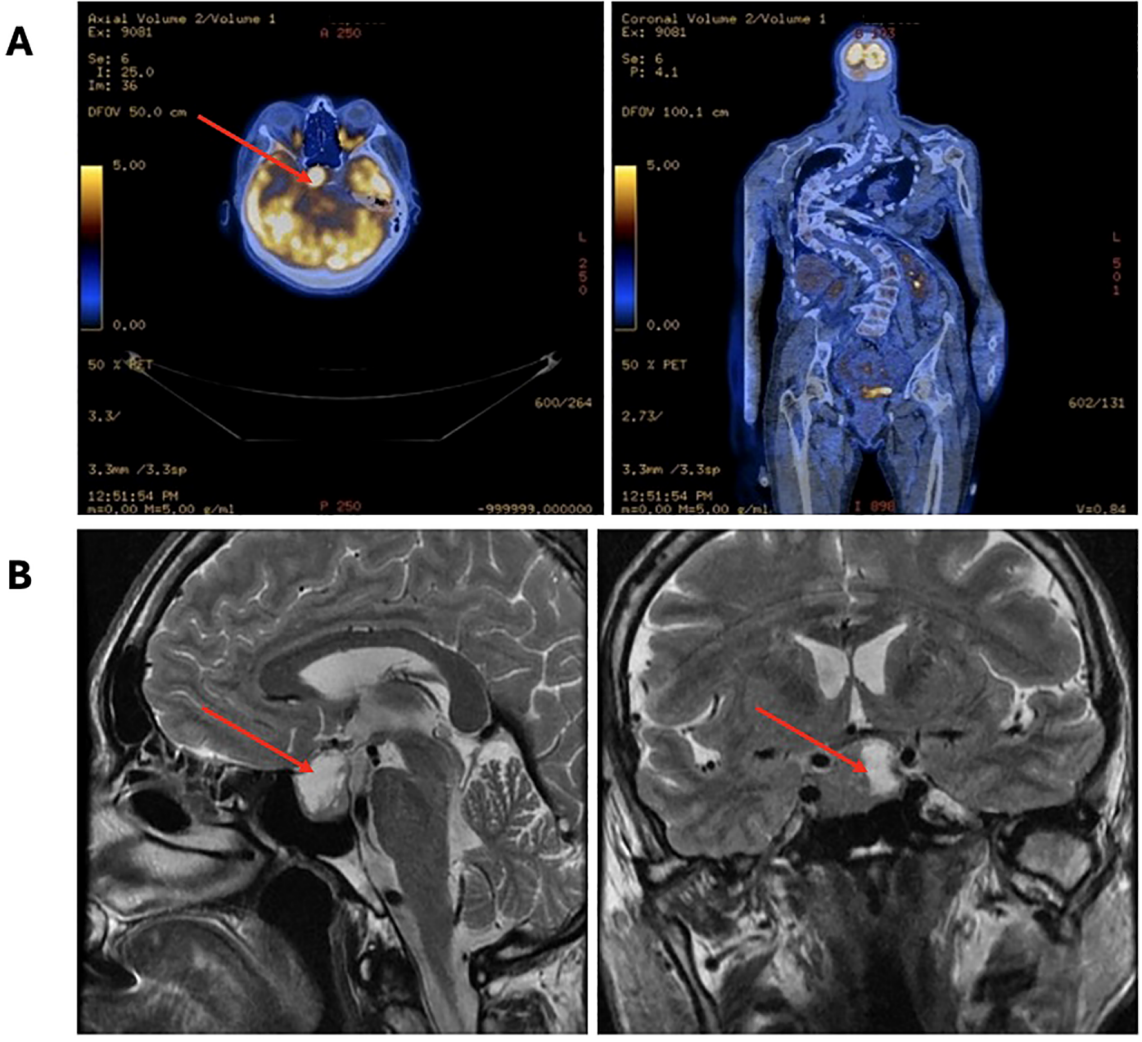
X-ray showing osteolytic lesions in the skeletal system, initially suspected to be metastatic in nature.
